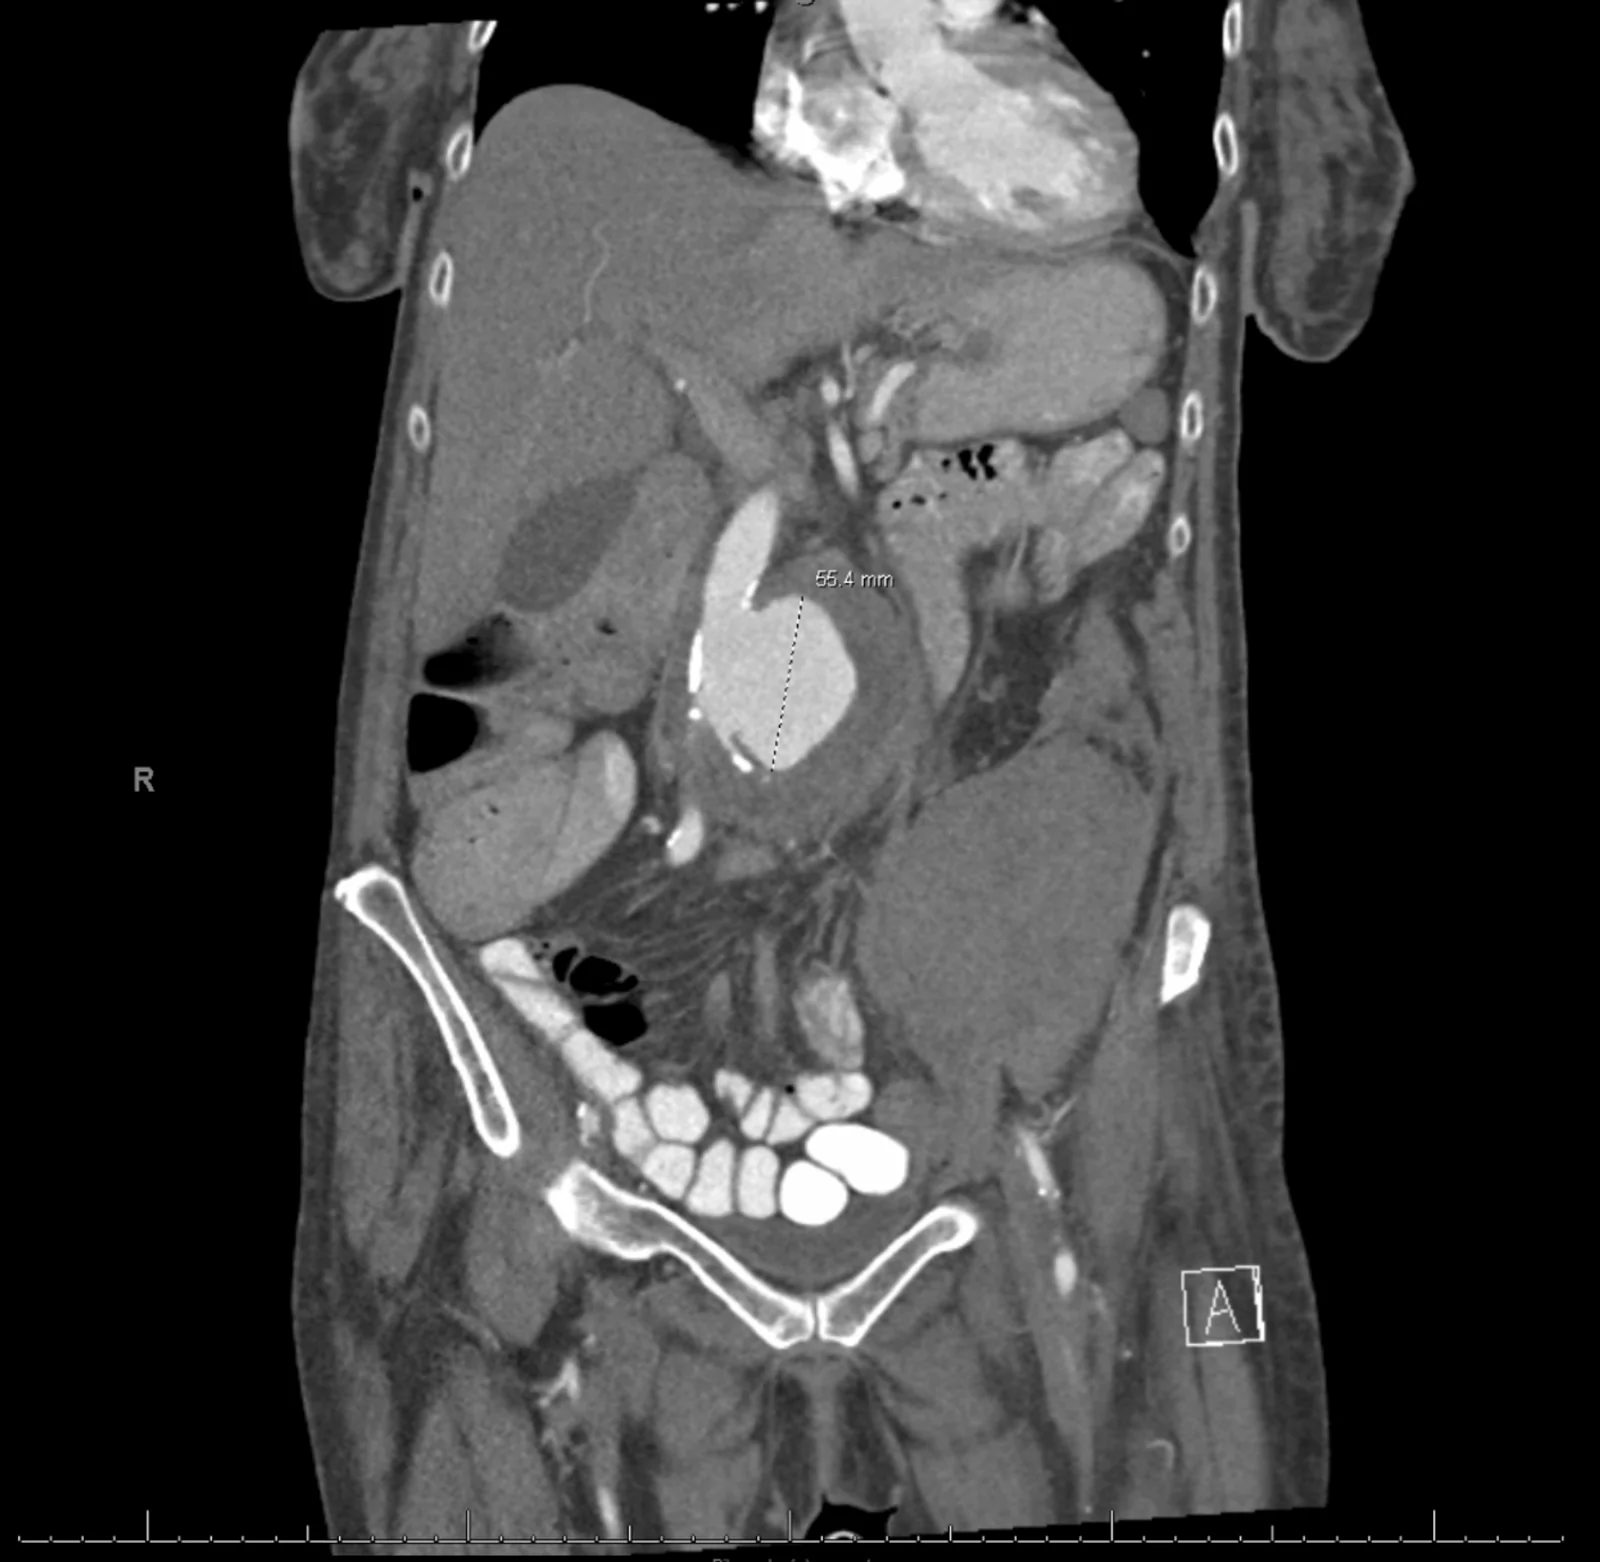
Coronal CTA demonstrating the infrarenal abdominal aortic aneurysm. The coronal image demonstrates the infrarenal abdominal aortic aneurysm, measuring approximately 5.5 cm on the displayed image. CTA: Computed tomography angiography.
